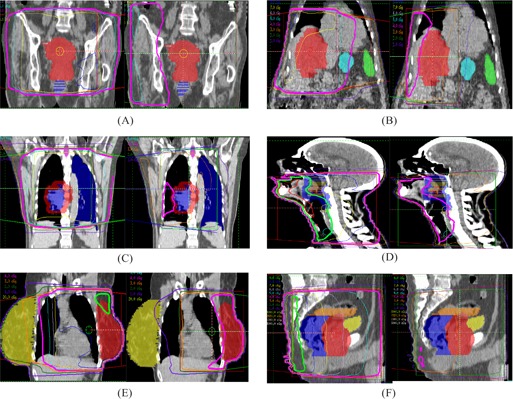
The two‐dimensional absolute dose distribution of sagital/coronal central slice is evaluated in various treatment sites—(A) pelvis, (B) abdomen, (C) lung, (D) head and neck, (E) breast, and (F) prostate—using megavoltage cone‐beam computed tomography images at 9 MUs (left panels) and orthogonal portal images at 4 MUs (right panels).
